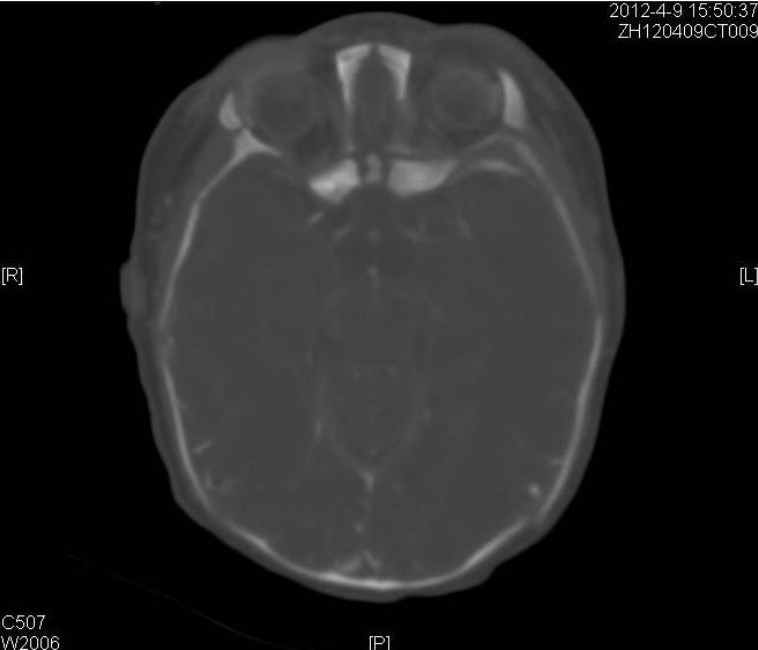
Figure 2: CT scan showing microphthalmia
